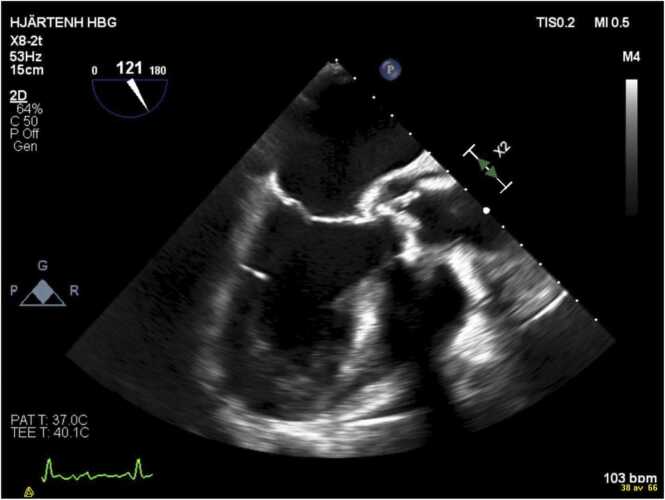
Transesophageal echocardiography of the patient on day 18 of hospitalization.
